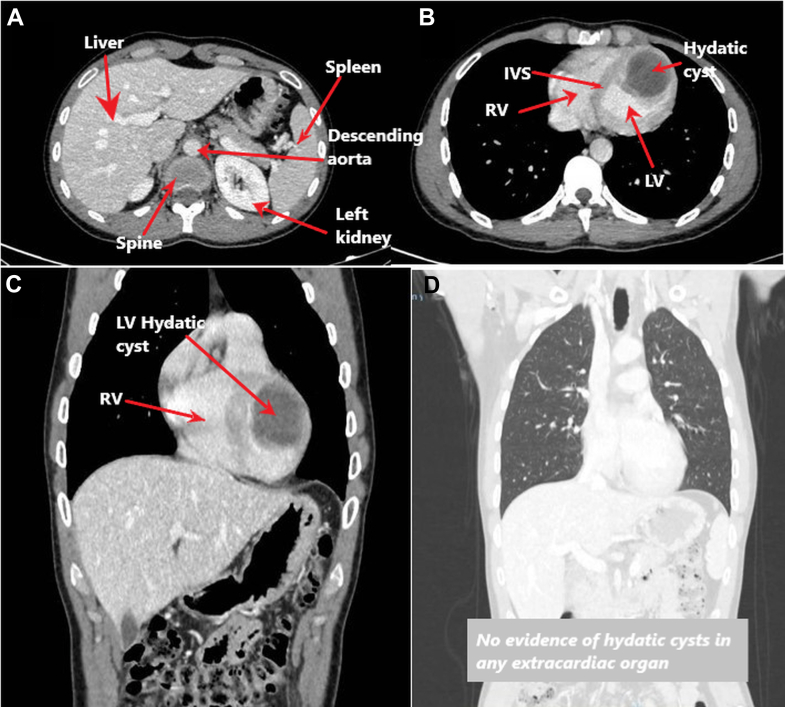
Preoperative Contrast-Enhanced CT Demonstrating Isolated Cardiac Hydatid Disease (A) No mediastinal lymphadenopathy, pulmonary nodules, or parenchymal abnormalities are identified. (B-C) Preoperative contrast-enhanced CT of the chest and abdomen showing a 40 x 36 mm multiloculated cystic mass located in the LV wall, radiologically consistent with a cardiac hydatid cyst. (D) Abdominal imaging reveals normal liver, gallbladder, biliary tree, pancreas, spleen, kidneys, adrenal glands, and mesenteric structures, with no evidence of hydatid cysts in any extracardiac organ. These findings confirm isolated cardiac hydatidosis with no other systemic involvement. CT = computed tomography; other abbreviations as in Figure 2.
